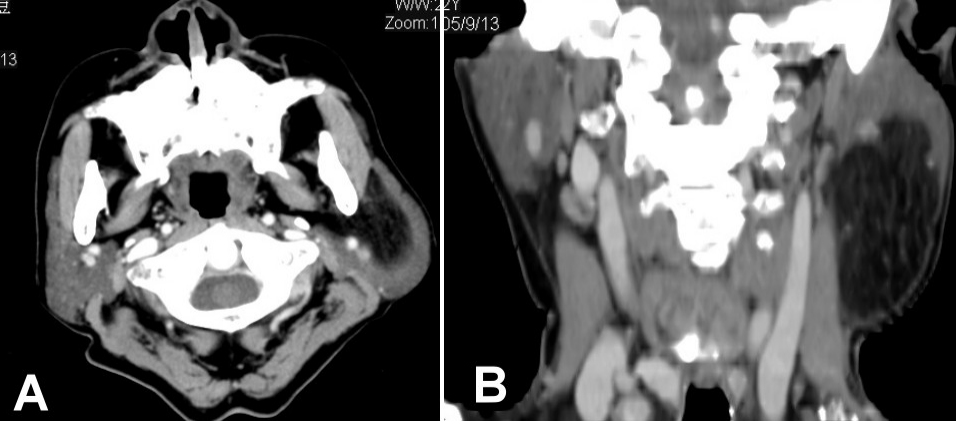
High-resolution post-contrast CT scans shows a spherical, well marginated, lower attenuation giant lipoma arising from left deep parotid lobe. (A) Axial view, the lipoma with rim of parotid gland overlaying lateral margin. (B) Reformatted coronal view, the lipoma is clearly defined from adjacent structures.
